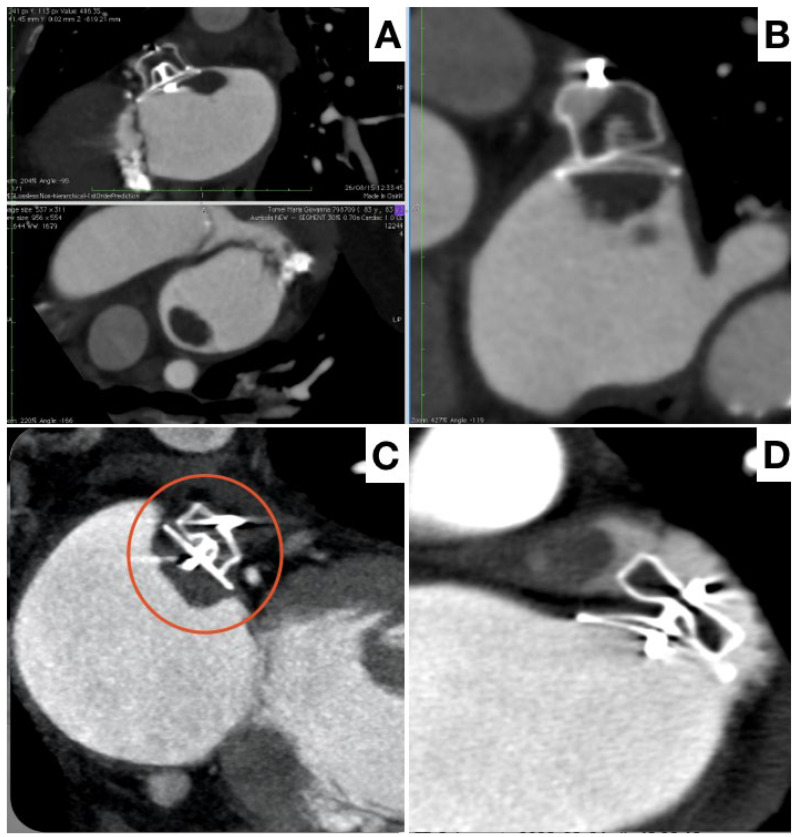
Different potential aspects of thrombosis at follow-up by CT. Pedunculated, hypermobile thrombus attached to the device (A), flat and stratified thrombus on the device (B,C red circle), presence of thrombus within unexcluded LAA (D).
